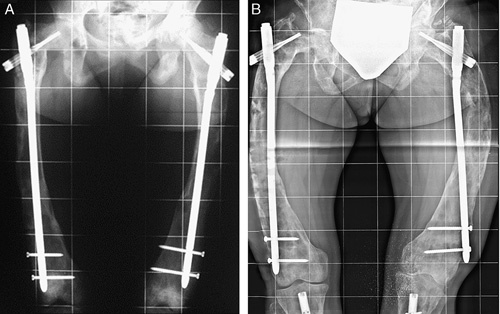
A, Standing anteroposterior radiograph of the pelvis and femurs of a 15-year-old patient with McCune-Albright syndrome, 2 years after her last bilateral intramedullary nailing. The patient was lost to follow-up but, 14 years later, she accepted to participate in the study. Radiographs (B) showed a severe recurrence of the femoral deformities with nail migration. She had a severe painful limp, but she was able to walk without any aid. Poor result.
